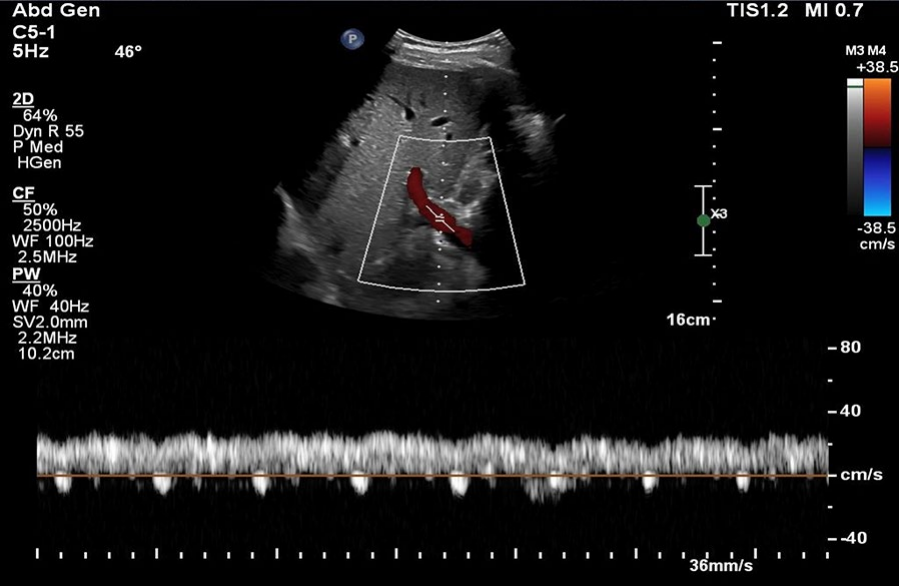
Pulsed-wave Doppler tracing of the PV. PV, portal vein
